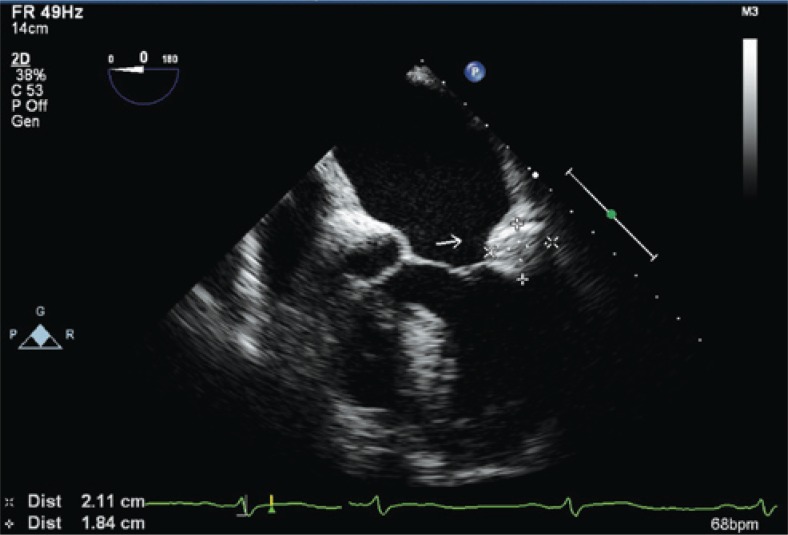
Transesophageal echocardiogram showing a heterogeneous mass and calcifications points located in posterior mitral valve annulus
